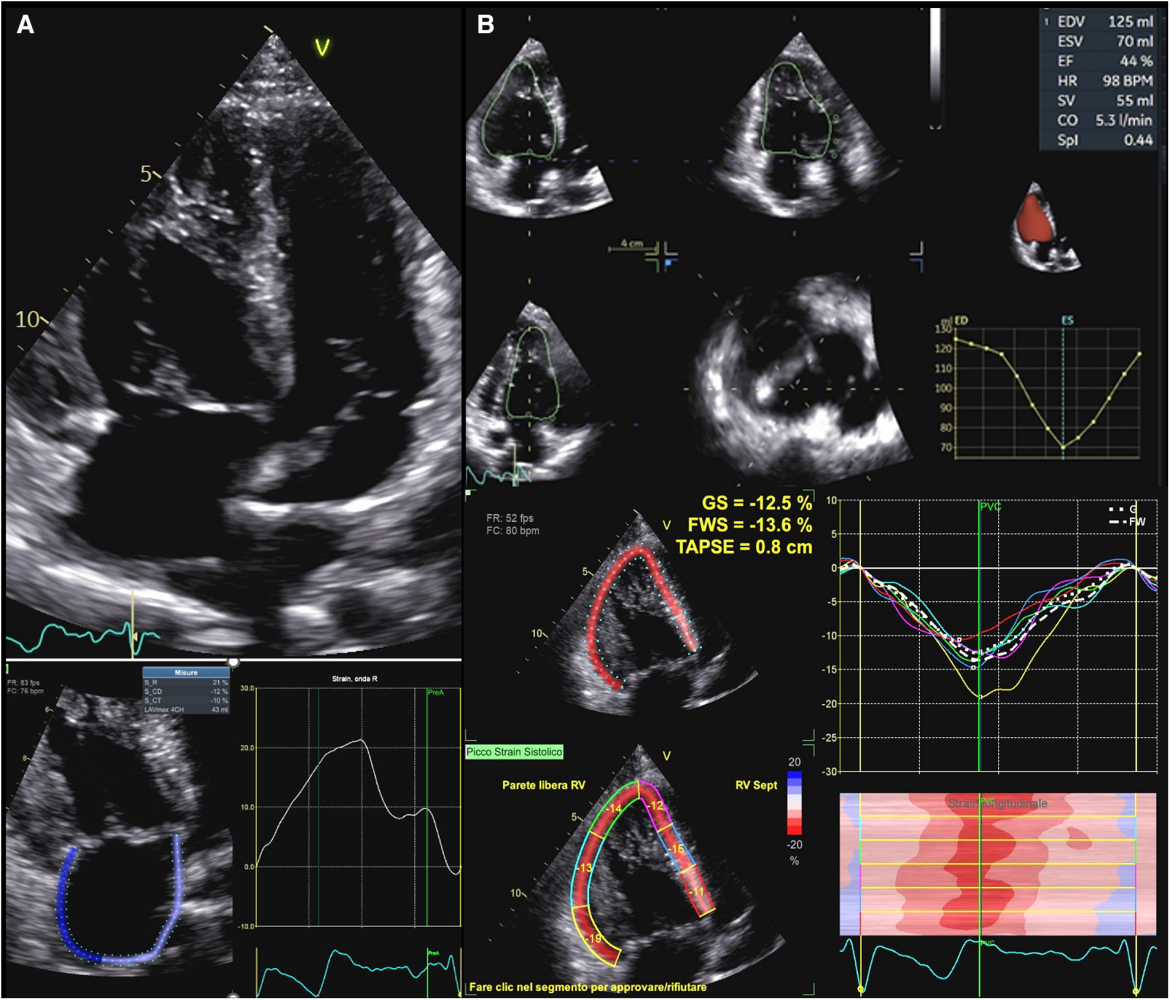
Functional assessment in systemic right ventricle physiologies. (A) Upper panel: apical 2D view in a patient with D-TGA after Senning operation. Lower panel: right atrial strain in the same patient. Atrial reservoir is impaired in this patient (21%). (B): Upper panel: sRV function as assessed by 3DE in a patient with ccTGA. Lower panel: GLS values in the same patient, which are impaired in all segments. D-TGA, D-transposition of great arteries; sRV, systemic right ventricle; 3DE, three-dimensional echocardiography; ccTGA, congenitally corrected transposition of great arteries.
